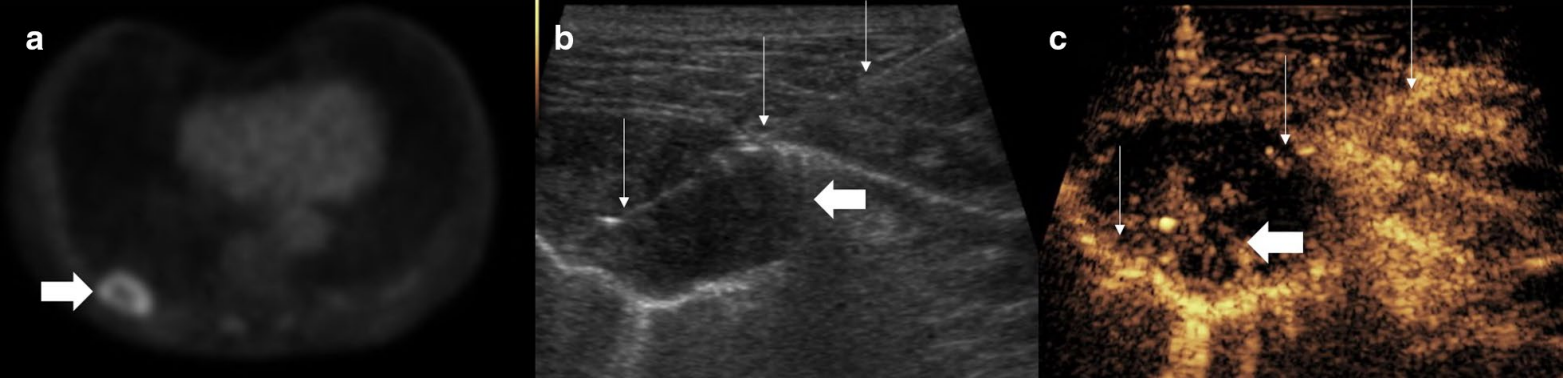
Patient with squamous cell carcinoma. a PET-CT shows a peripherally avid lesion with no central avidity (arrow). b, c Simultaneous B mode and CEUS images as the biopsy is performed. B mode demonstrates a hypoechoic lesion (thick arrow) with the biopsy needle within the lesion (thin arrow). The contrast image (c) shows the biopsy (thin arrows) is taken from the deep area of enhancement and therefore viable tissue (thick arrow)
